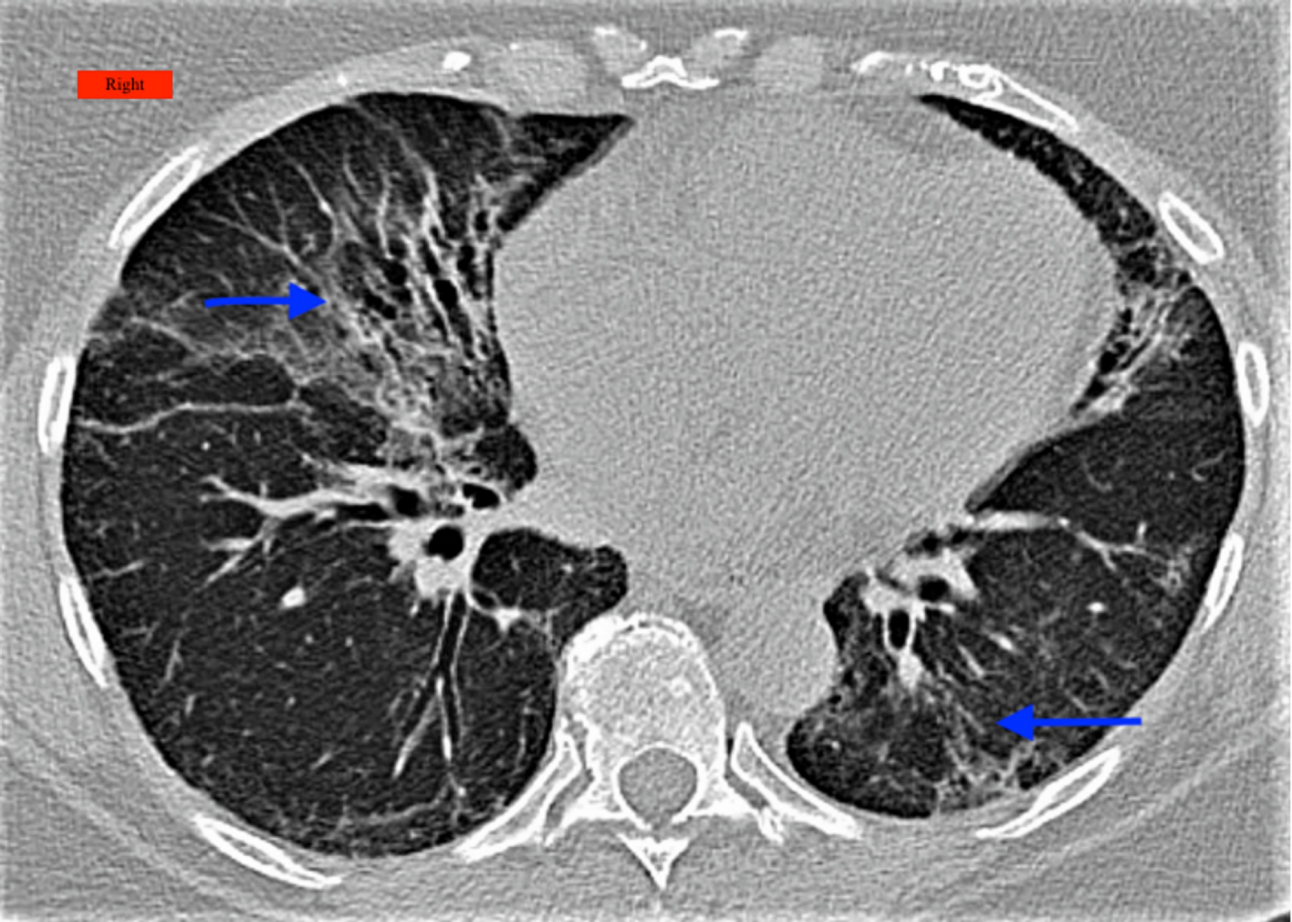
Computed tomography chest showing interlobular and intralobular septal thickening and some ground-glass opacities (arrows) Right: Indicates the right side of the patient
